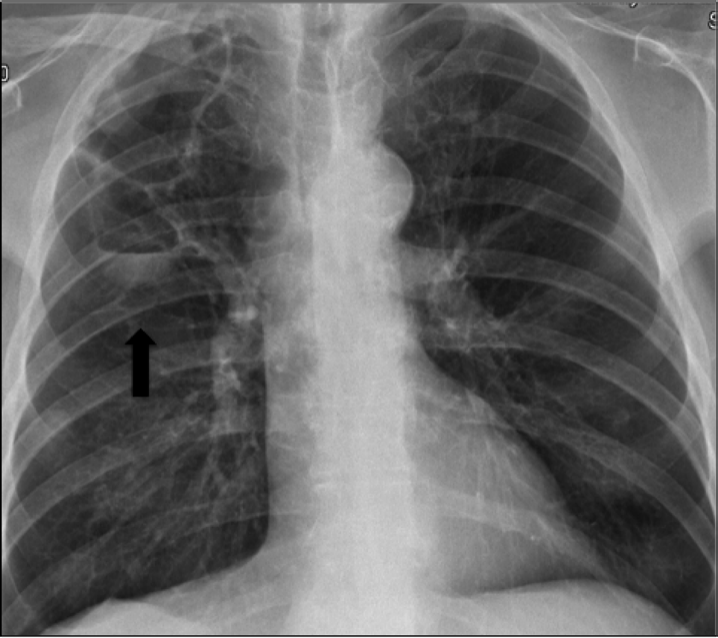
Pneumatocele enlargement detected in chest X-ray *(black arrow)* (Case 2)
